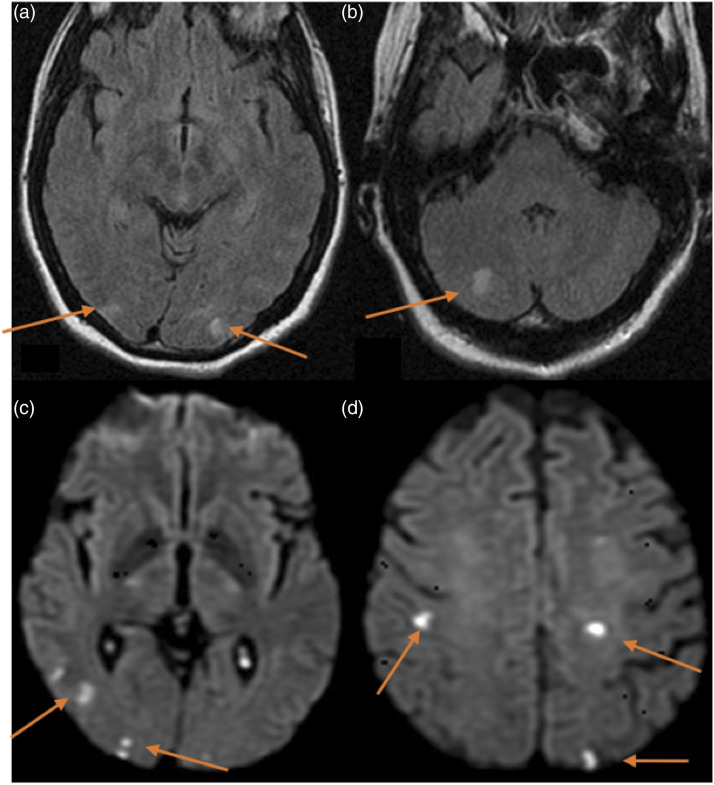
Multiple cerebral emboli case. A 58-year-old male patient with chronic obstructive pulmonary disease underwent double-LT. He presented an acute motor deficit in the right superior extremity 3 years after the transplantation. Besides, atrial fibrillation was recently diagnosed. Figures: axial MRI FLAIR sequences (a and b) and MRI diffusion-weighted sequences (c and d). Multiple cortical and subcortical hyperintense foci in occipito-parietal lobes (arrows in a and b). Some of these foci show restricted diffusion (arrows in c and d) related to acute infarctions.
